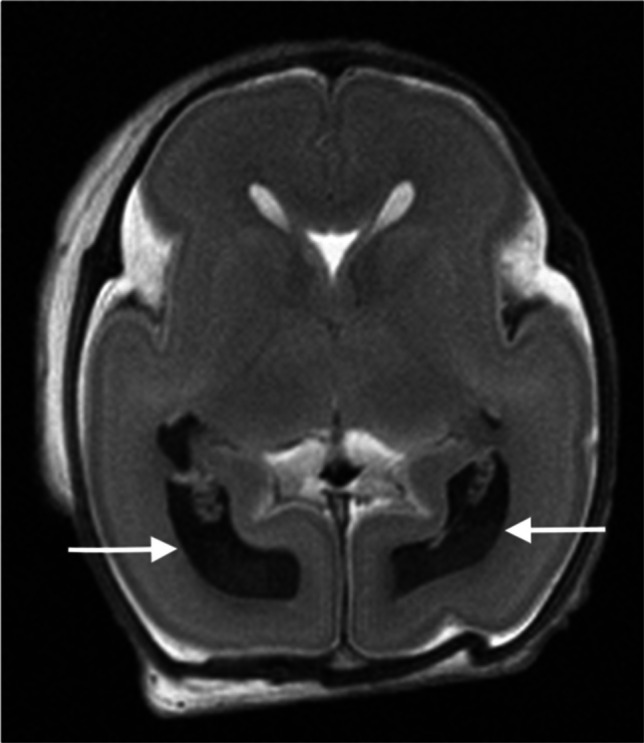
Axial T2-weighted postmortem magnetic resonance image in a 25-week-old female fetus showing classical intraventricular hemorrhage (*arrows*). Adapted with permission [33]
